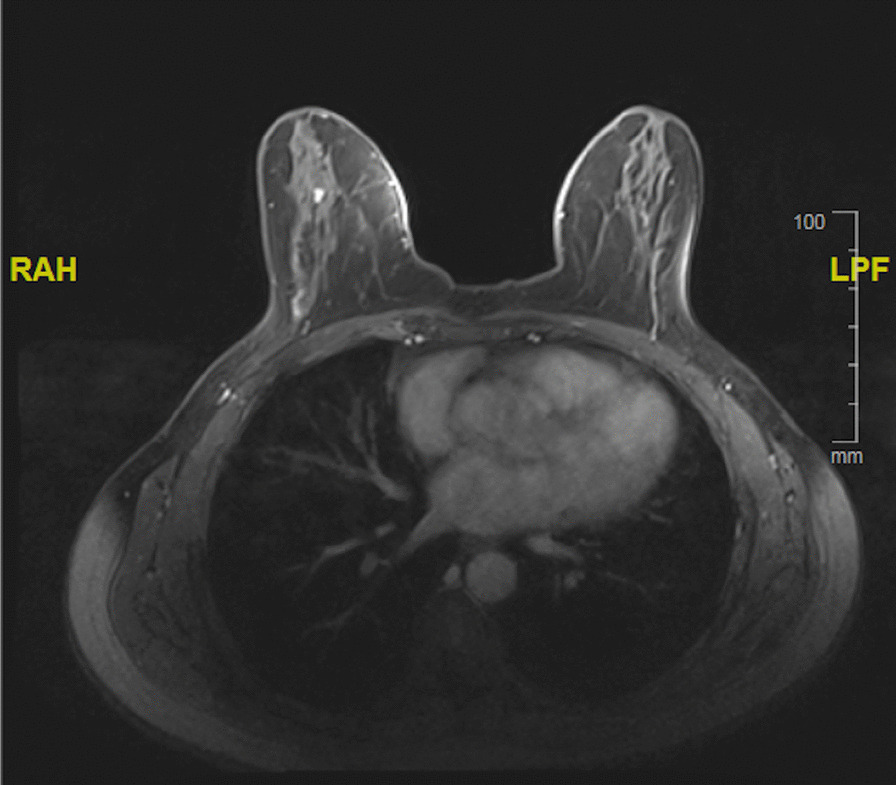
Breast magnetic resonance imaging showing non mass-like clumped progressive enhancement of microcalcification in the right breast. RAH Right, LPF Left
